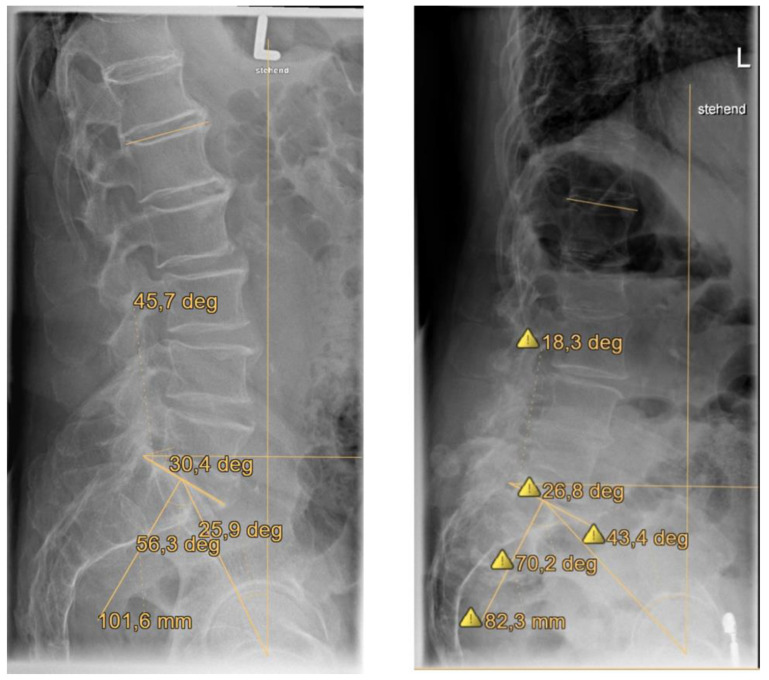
Spinopelvic parameters of LL, PT, SS and PI were analyzed for all patients. Measurement was conducted in a standing lateral radiograph of the spine and pelvis in which the spine from T12 to S1 and both femoral heads were visible.
